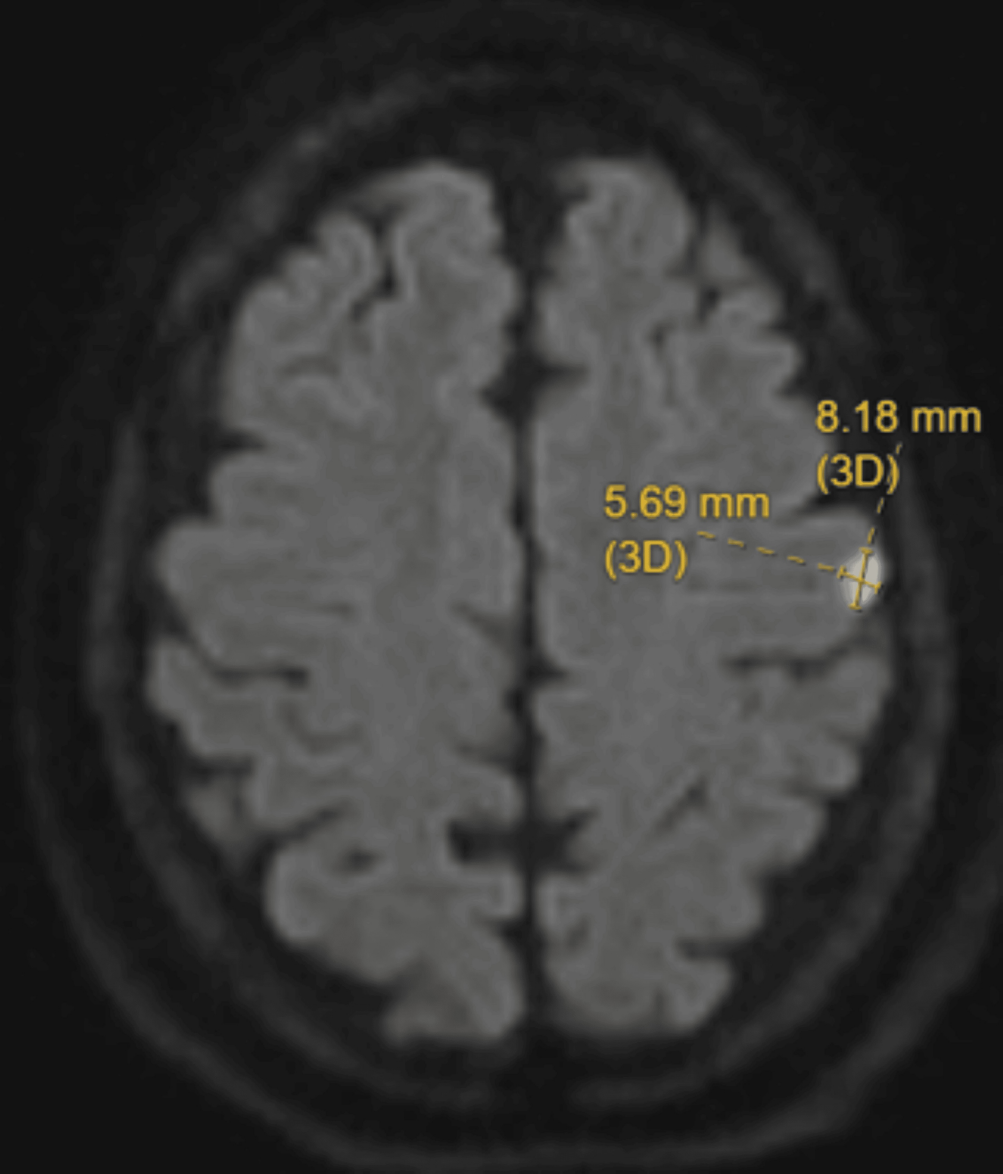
MRI DWI sequence showed a 5.69 x 8.18 mm acute ischemic stroke in the left frontal lobe anterior to the left central sulcus DWI: Diffusion-weighted imaging
